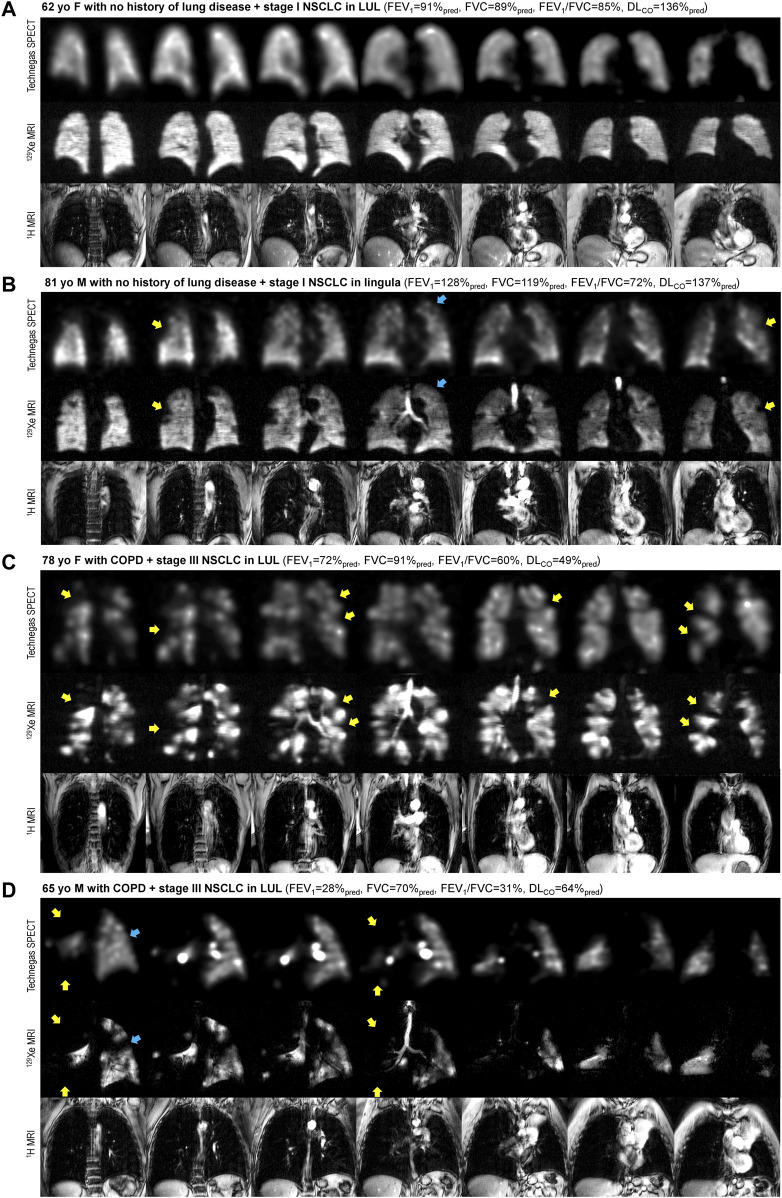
Comparison of ventilation visualized by Technegas SPECT and hyperpolarized ^129^Xe MRI. Anatomically matched coronal Technegas SPECT, ^129^Xe MRI, and ^1^H MRI slices for four representative participants. Select examples of spatially concordant and discordant ventilation defects are highlighted by yellow and blue arrows, respectively. (A) 62-year-old female with necrotizing granuloma in left upper lobe and no history of lung disease. Technegas SPECT: VDP_T_ = 12%*, VDP_K_ = 5%; ^129^Xe MRI: VDP_T_ = 22%, VDP_K_ = 1%*; FEV_1_ = 91%_pred_, FVC = 89%_pred_, FEV_1_/FVC = 85%, DL_co_ = 136%_pred_. (B) 81-year-old male with stage I NSCLC in lingula and no history of lung disease. Technegas SPECT: VDP_T_ = 28%*, VDP_K_ = 14%; ^129^Xe MRI: VDP_T_ = 18%, VDP_K_ = 4%*; FEV_1_ = 128%_pred_, FVC = 119%_pred_, FEV_1_/FVC = 72%, DL_CO_ = 137%_pred_. (C) 78-year-old female with stage III NSCLC in left upper lobe and concomitant COPD. Technegas SPECT: VDP_T_ = 47%*, VDP_K_ = 20%; ^129^Xe MRI: VDP_T_ = 31%, VDP_K_ = 19%*; FEV_1_ = 72%_pred_, FVC = 91%_pred_, FEV_1_/FVC = 60%, DL_CO_ = 49%_pred_. (D) 65-year-old male with stage III NSCLC in left upper lobe and concomitant COPD. Technegas SPECT: VDP_T_ = 84%*, VDP_K_ = 52%; ^129^Xe MRI: VDP_T_ = 37%, VDP_K_ = 48%*; FEV_1_ = 28%_pred_, FVC = 70%_pred_, FEV_1_/FVC = 31%, DL_CO_ = 64%_pred_. COPD = chronic obstructive pulmonary disease; FEV_1_ = forced expiratory volume in one second; FVC = forced vital capacity; DL_CO_ = diffusing capacity for carbon monoxide; LUL = left upper lobe; NSCLC = non-small cell lung cancer; %_pred_ = percent of predicted value. *Thresholding (VDP_T_) and k-means clustering (VDP_K_) methods previously optimized and validated for Technegas SPECT and ^129^Xe MRI, respectively.
